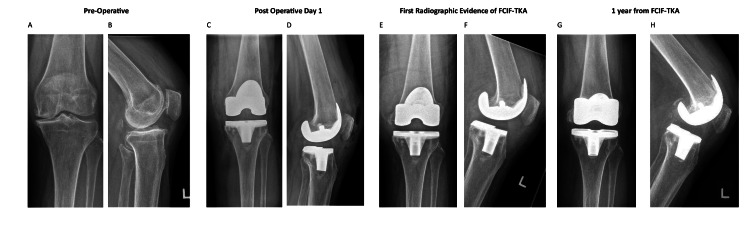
Patient 2 AP and lateral knee FCIF-TKA radiographs A: prepperative AP film, B: preoperative lateral film, C: postoperative day 1 AP film, D: postoperative day 1 lateral film, E: first radiographic evidence of FCIF-TKA AP film, F: frst radiographic evidence of FCIF-TKA lateral film, G.: one year from FCIF-TKA AP film, H: one year from FCIF-TKA lateral film AP: anterior-posterior, FCIF-TKA: femoral condyle insufficiency fractures following total knee arthroplasty
